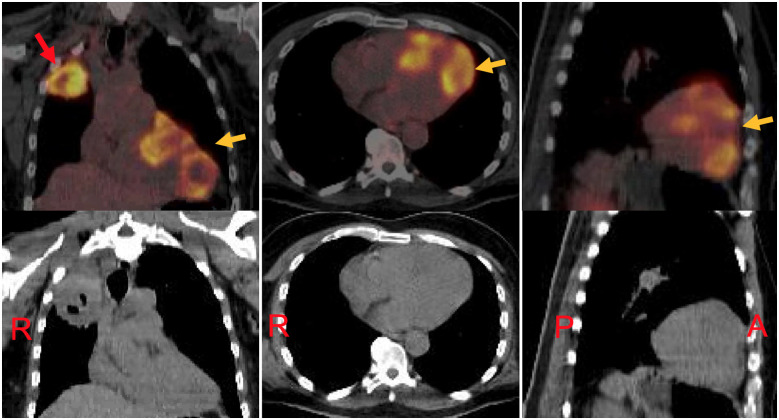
An 18F-fluorodeoxyglucose*-*positron emission tomography-computed tomography (FDG-PET-CT) imaging showing hypermetabolic masses consistent with myocardial metastases (orange arrows) and hypermetabolic mass within right upper lung lobe consistent with primary malignancy (red arrow). Computed tomography images are shown in lower panel. A, anterior; P, posterior; R, right.
